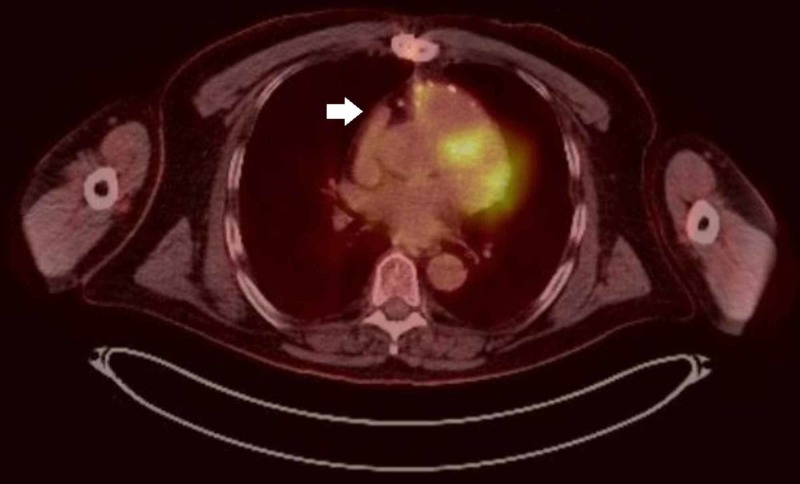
Post-treatment changes in the right ventricular wall without discrete F-fluorodeoxyglucose (FDG) avid mass (arrow). No hypermetabolic chest mass, fluid collection, or lymphadenopathy.
